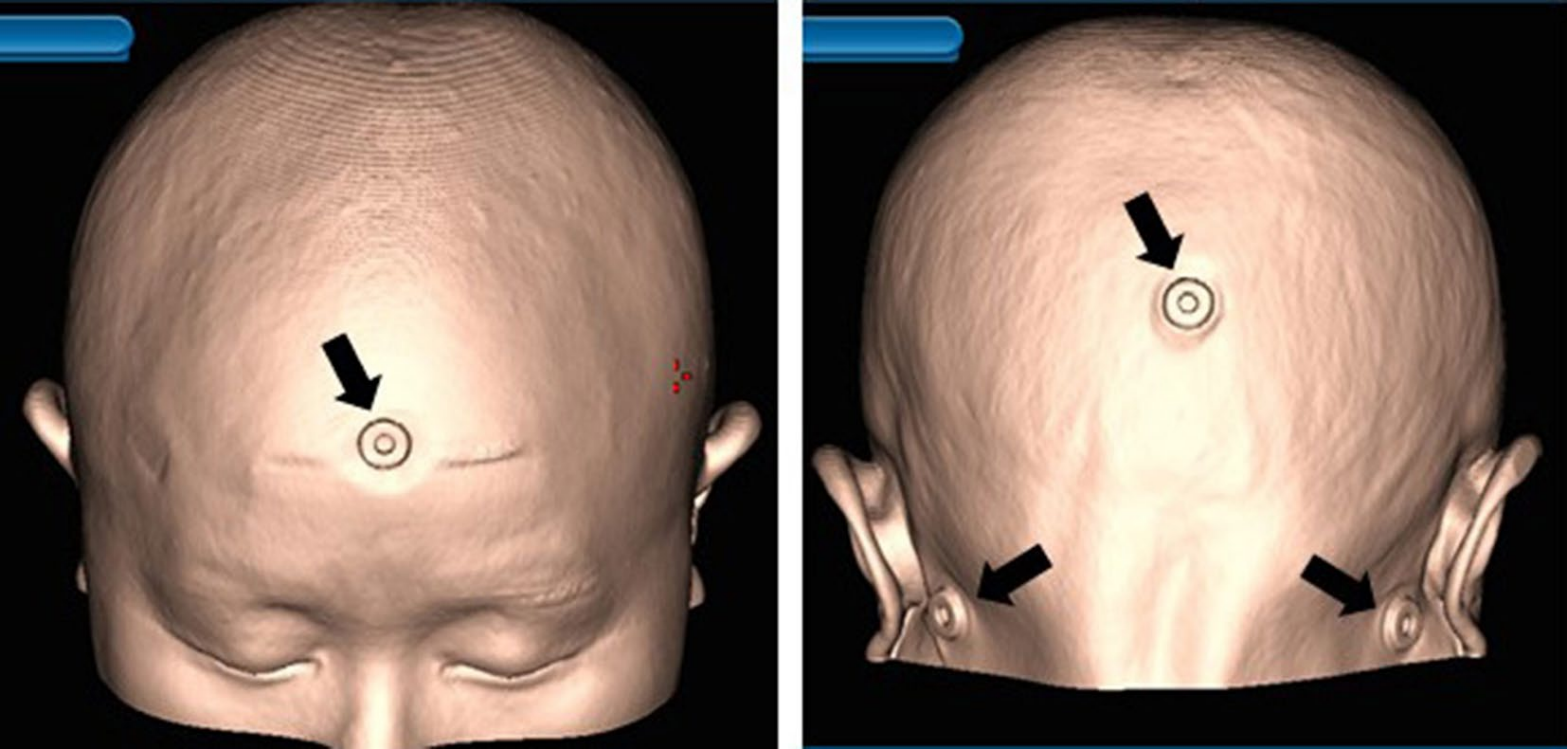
Fiducial markers were attached to the head as landmarks for the front, the back, the right, and left. A three-dimensional reconstruction using computed tomography shows that four fiducial markers (arrows) were implanted in the patient’s forehead (nasion), occipital region (inion), and bilateral retroauricle regions (mastoid process).
